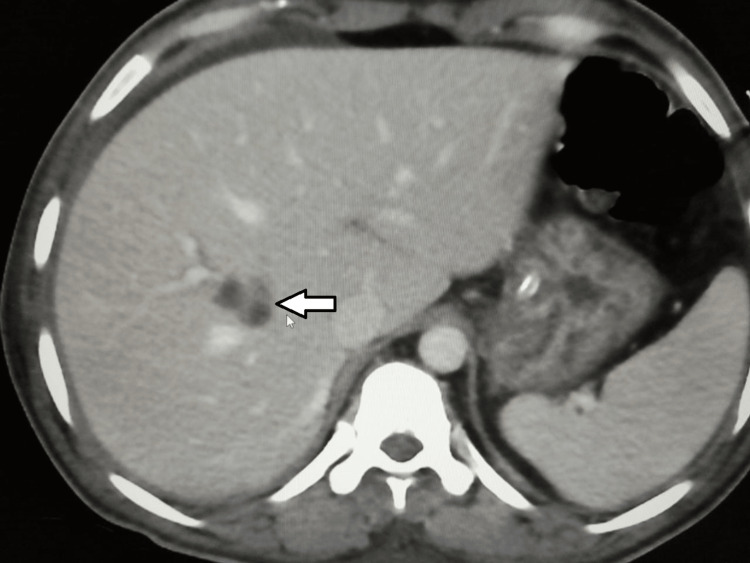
Abdominal CT scan The scan shows a small septated cystic lesion in segment 8/1 of the liver (white arrow).
